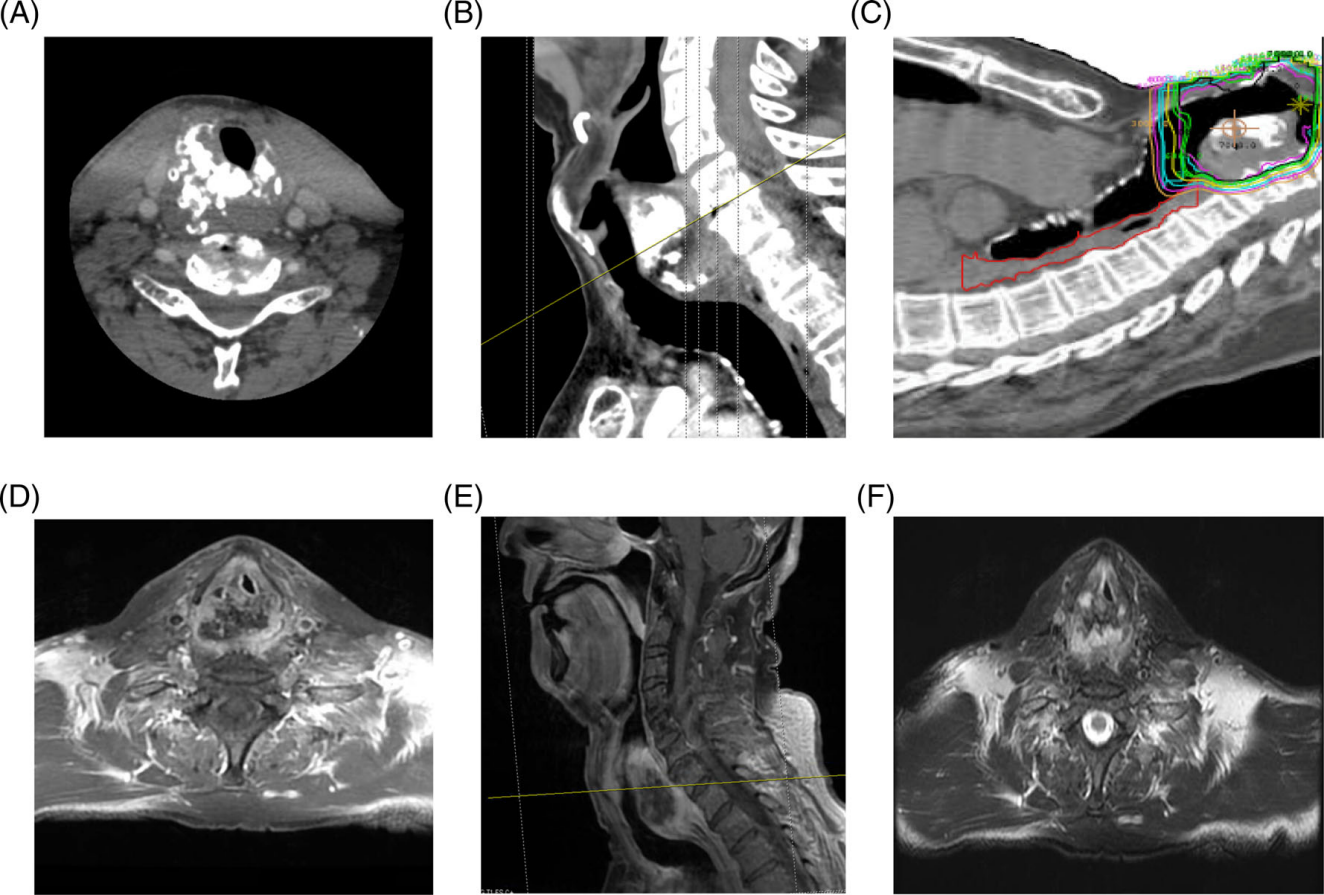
Representative (A) axial and (B) sagittal CT images of case 4's chondrosarcoma at initial presentation. Dose distribution for radiotherapy plan (C). Isodose lines (cGy): red = 7600; black = 7000; green (inner) = 6860; green (outer) = 6650; yellow = 6000; teal = 5000; purple = 4000; orange = 3000. Representative (D) axial, (E) sagittal T1‐weighted and (F) axial T2‐weighted post‐contrast MRI images demonstrating chondrosarcoma 5 months after completion of radiotherapy
